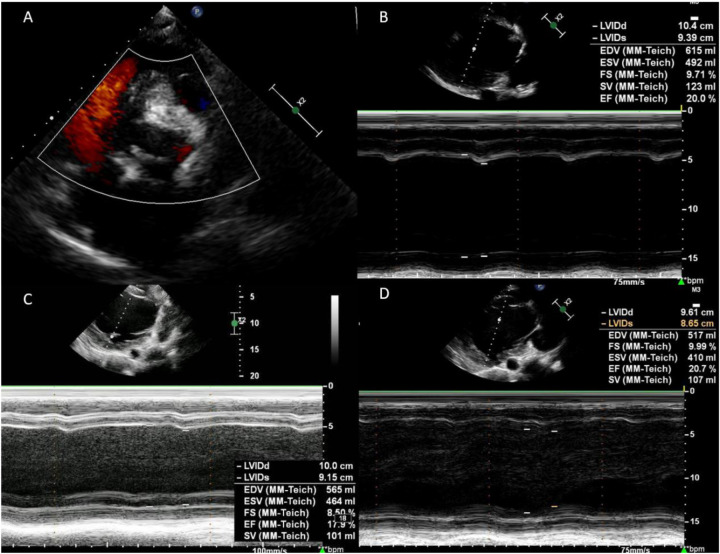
(A) Postoperative aortic short-axis view showing the occluder in a satisfactory position with no residual shunt on color Doppler. (B) Postoperative LVEDD 104 mm; (C) LVEDD 100 mm at 2 months postoperatively; (D) LVEDD 96 mm at 6 months postoperatively.
